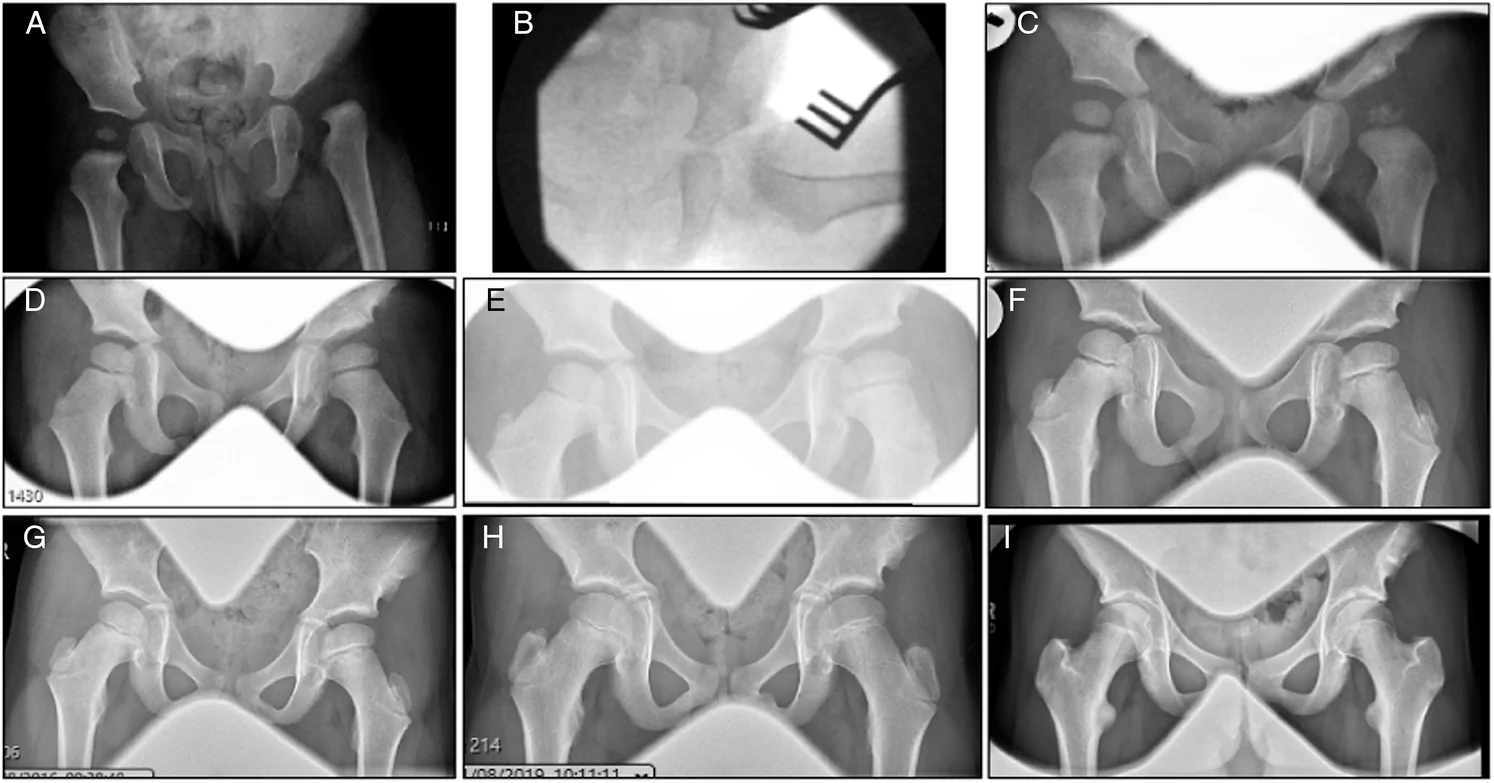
Serial radiographs following left hip open reduction and growth-stimulating minimal acetabuloplasty, in a girl who is now 14 years old and at skeletal maturity. (A) Preoperative showing IHDI 3 hip with an AI of 41 degrees, compared with 23 degrees on the normal right hip. (B) Intraoperative fluoroscopy at 11 months of age, showing the pellet in the upper outer edge of the acetabulum. (C–H) Progressive development of the hip and the AI at 1, 2, 3, 4, 5, and 8 years following surgery. (I) Thirteen years postoperative: AI 5 degrees and symmetrical; CEA 43 degrees; Severin 1; asymptomatic with normal, symmetrical clinical examination.
